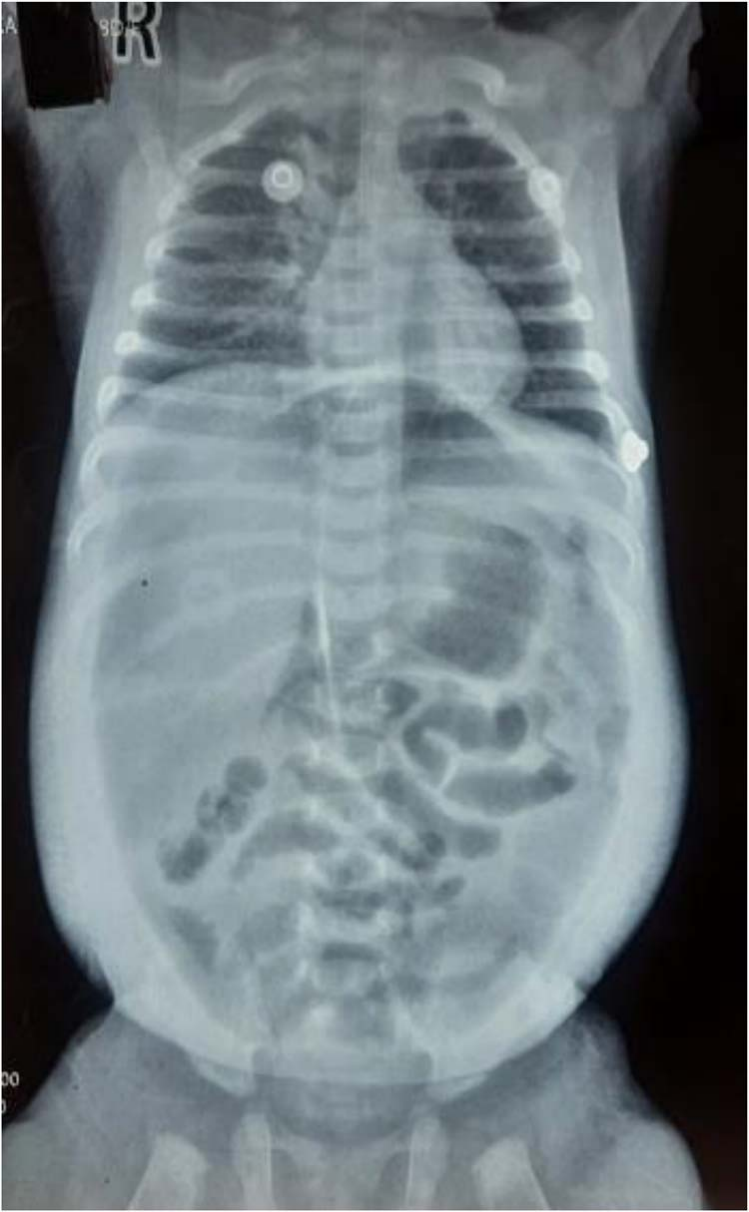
Supine X-ray showing chest and abdomen.
